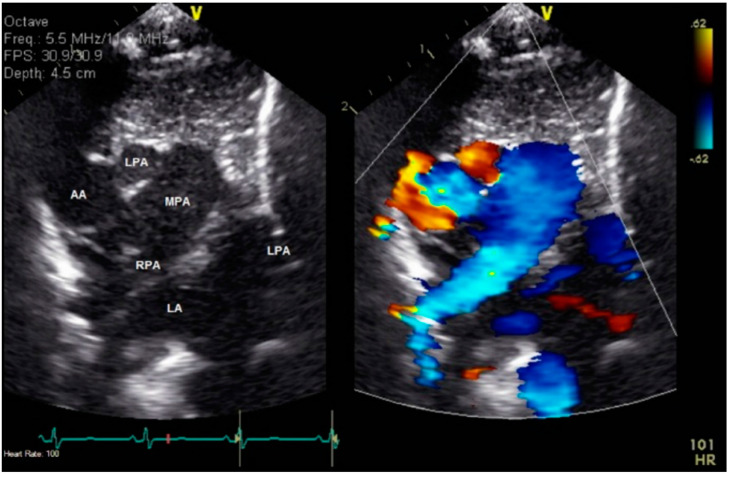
Echocardiography in the high-right parasternal short-axis view. The left panel is a two-dimensional image while the right panel is color interrogation of the same image. The pulmonary trunk is seen giving rise to a right pulmonary artery, without evidence of another vessel branching from the pulmonary trunk. The ascending aorta is visualized posterior and rightward, and there is an additional structure noted between the ascending aorta and the pulmonary trunk that appears to course anteriorly from the ascending aorta. This was confirmed by additional imaging to be the left pulmonary artery arising from the ascending aorta. The left pulmonary artery can be seen arising from the ascending aorta and then coursing leftward, crossing the pulmonary trunk and the right pulmonary artery in its course.
